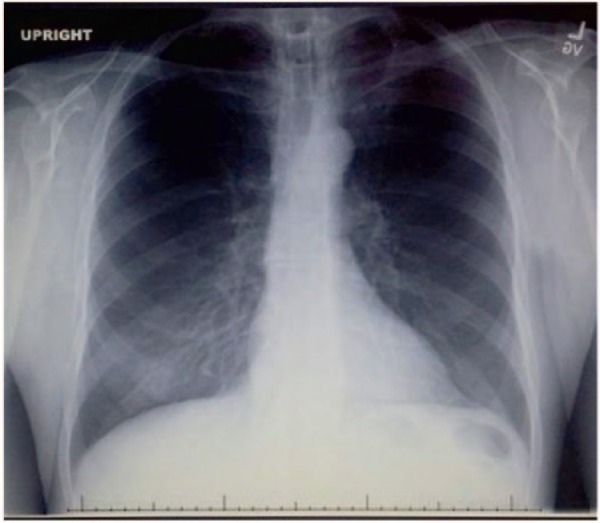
Chest X-ray 1 week after discharge—complete resolution of pleural effusions.
